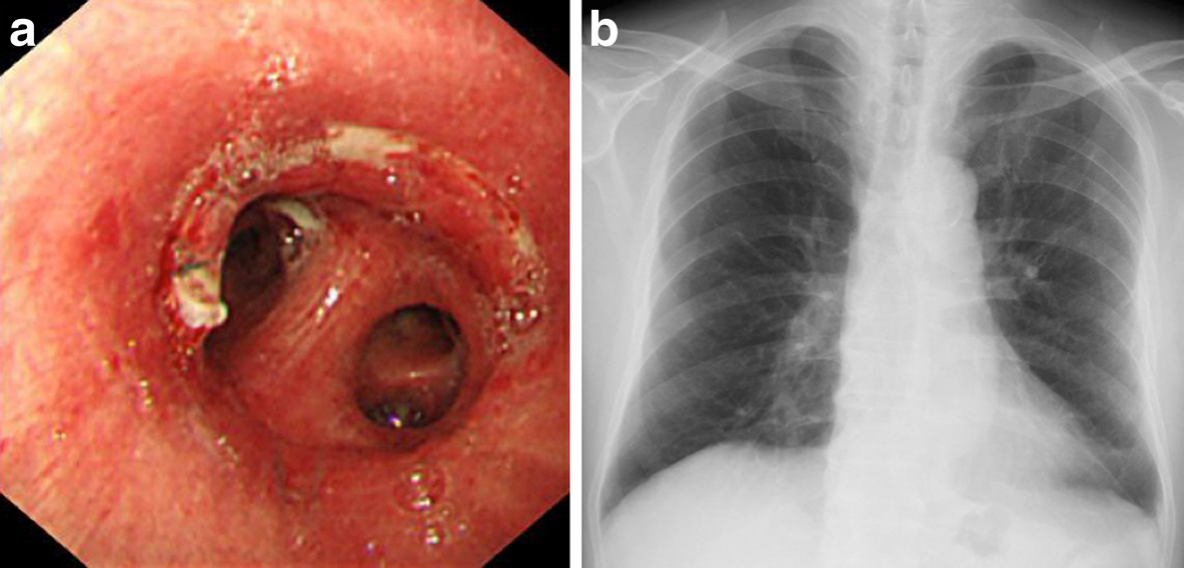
Postoperative examination. a, Postoperative bronchoscopy showed that the anastomosis was intact. b, Postoperative chest X-ray revealed good lung expansion bilaterally.
